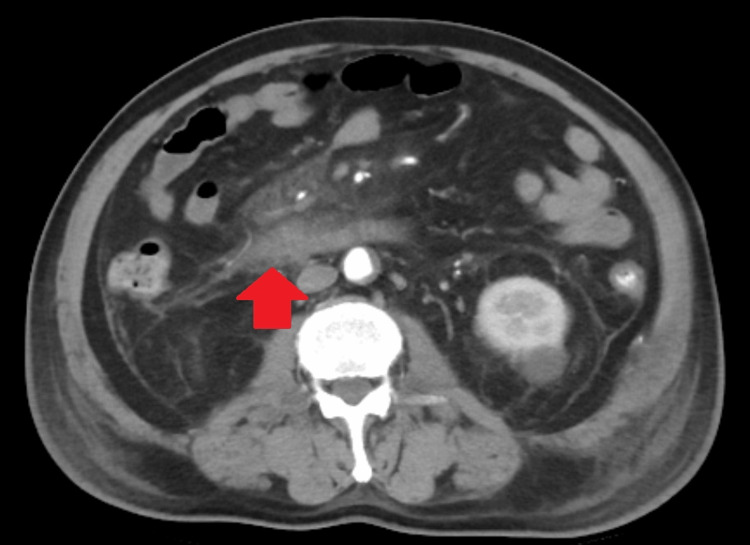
Contrast-enhanced abdominal CT indicating the spread of inflammation in the abdominal cavity (red arrows)
